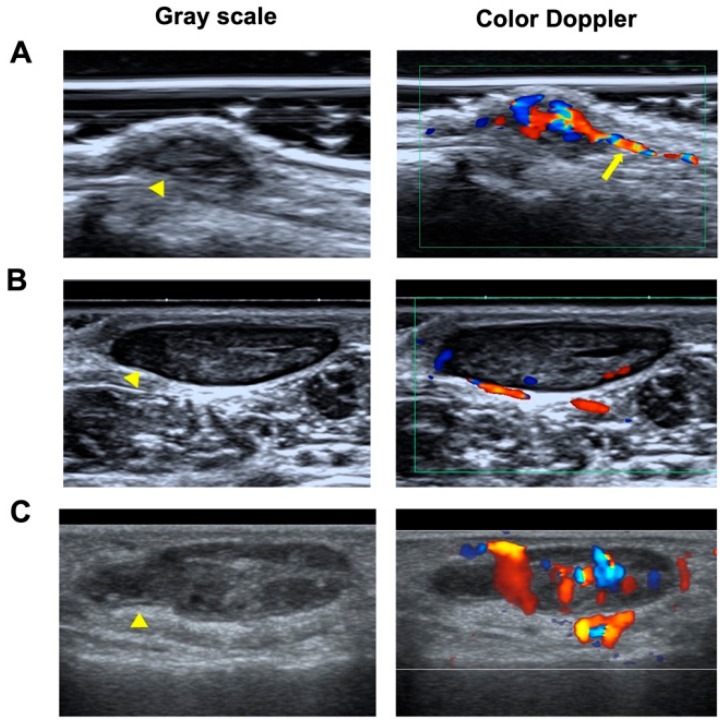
Representative gray scale and color Doppler ultrasonograms of NSGT, ALM, and hemangioma. (A) Gray-scale ultrasound images obtained using high-frequency ultrasound show a hypoechoic mass measuring 7 × 3 mm in the subcutaneous tissue in the right arm of a 56-year-old man (yellow arrowhead). Color Doppler ultrasound reveals a hypervascular lesion (grade 3) with a vascular stalk sign (yellow arrow). (B) A hypoechoic mass measuring 15 × 6 mm in the left foot of a 31-year-old man (yellow arrowhead), with vascularity of grade 1. (C) A hypoechoic mass measuring 19 × 6 mm on the right finger of a 36-year-old woman (yellow arrowhead), with vascularity of grade 3.
